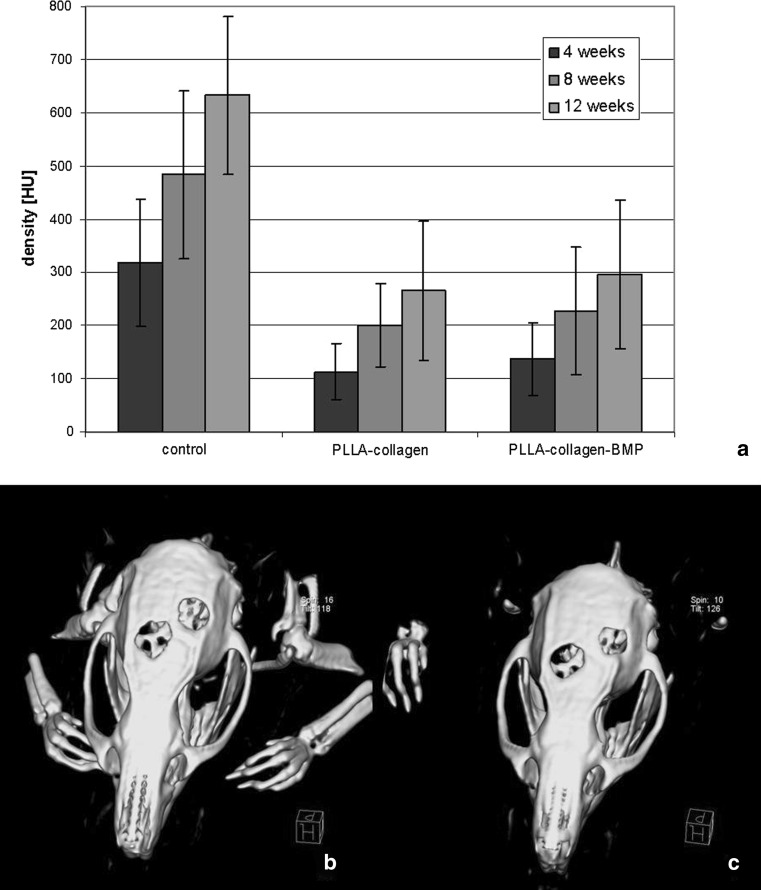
Influence of nanofiber implant on defect calcification Radiological density [HU] of defect areas measured by cranial CT-scans (a), and 3D reconstructions of cranial CT-scans (b), PLLA-collagen type I (c), and PLLA-collagen type I/BMP-2, each 4 weeks after implantation
